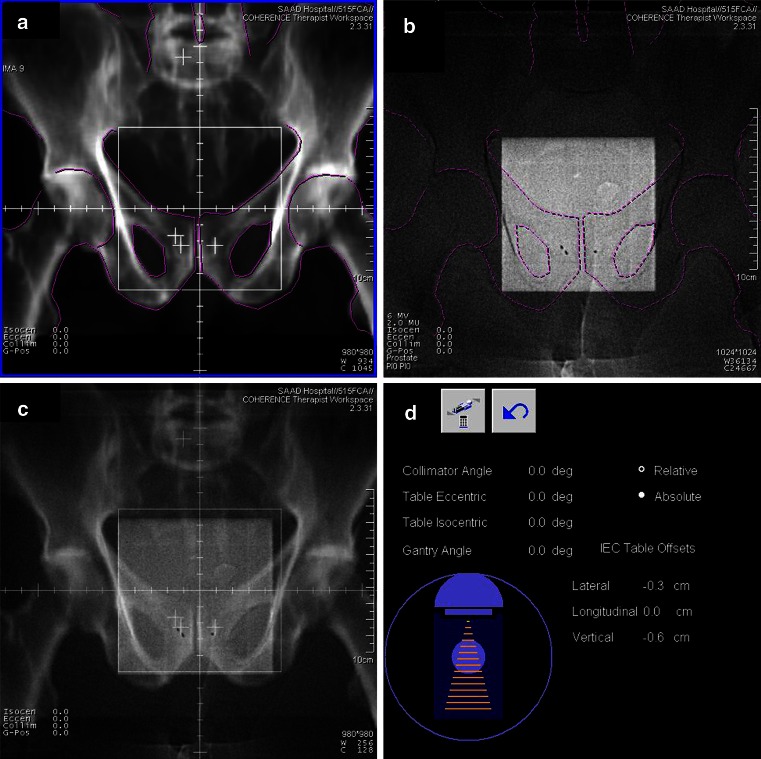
Online verification of bony landmarks and implanted fiducial prostate markers using megavoltage X-rays and electronic portal imaging device (*EPID*). a Digital reconstructed radiograph (*DRR*) generated by the treatment planning system. The position of the three fiducial prostate markers is marked with crosses. b Portal image (double-exposed) obtained immediately before the radiotherapy fraction using the EPID. The *three dots* represent the fiducial prostate markers. c Fused images of a and b. d Calculated deviation of the compared structures of images a and b
